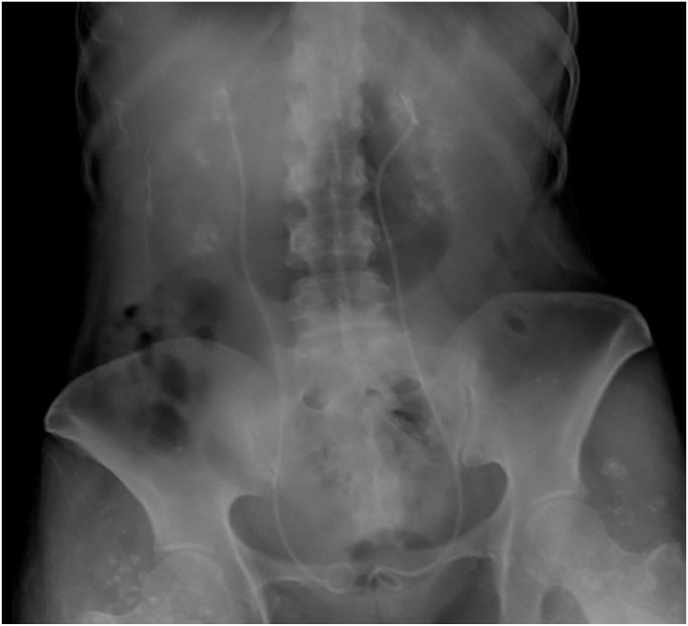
KUB X-ray showing bilateral nephrocalcinosis and the two double-J catheters in place.
